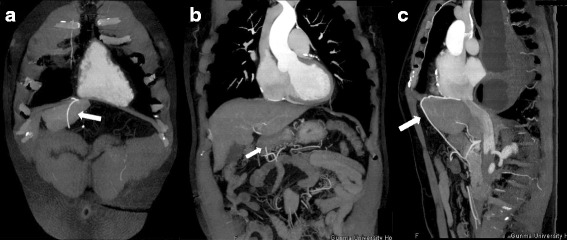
Three-dimensional CT findings. It is running through the front of the duodenal bulb and ventral of the hepatic lateral segment and going toward the back side beyond the diaphragm and reach to the RCA. a, b Coronal view. c Sagittal view. *White arrow* indicates the right gastroepiploic artery
